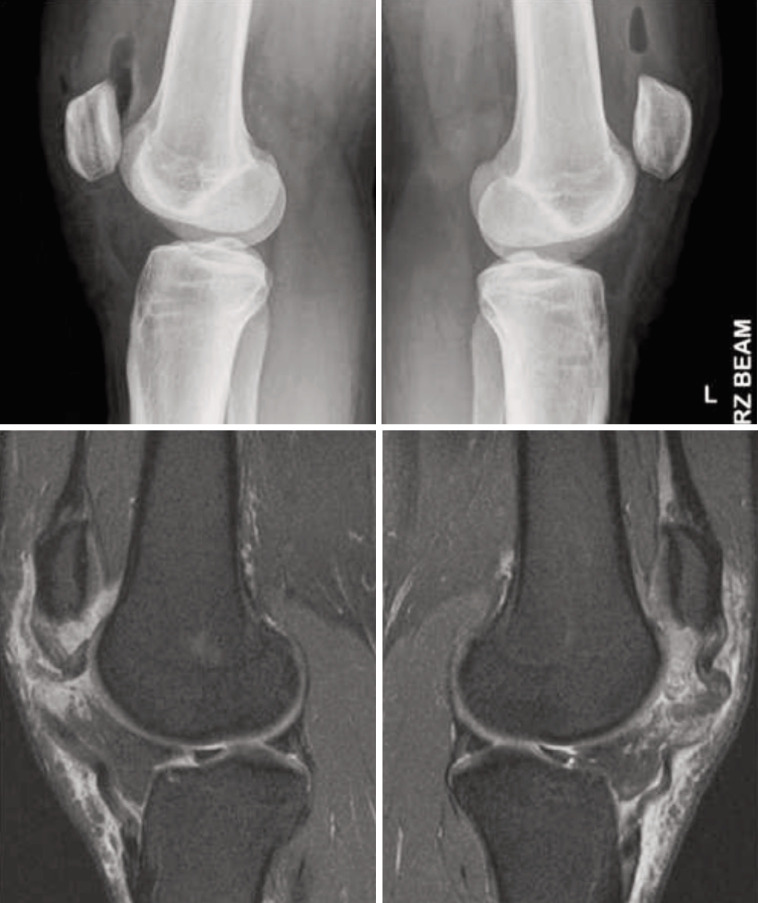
Bilateral lateral radiographs of knees showing patella alta and MRI scans of complete rupture of patella tendons.
